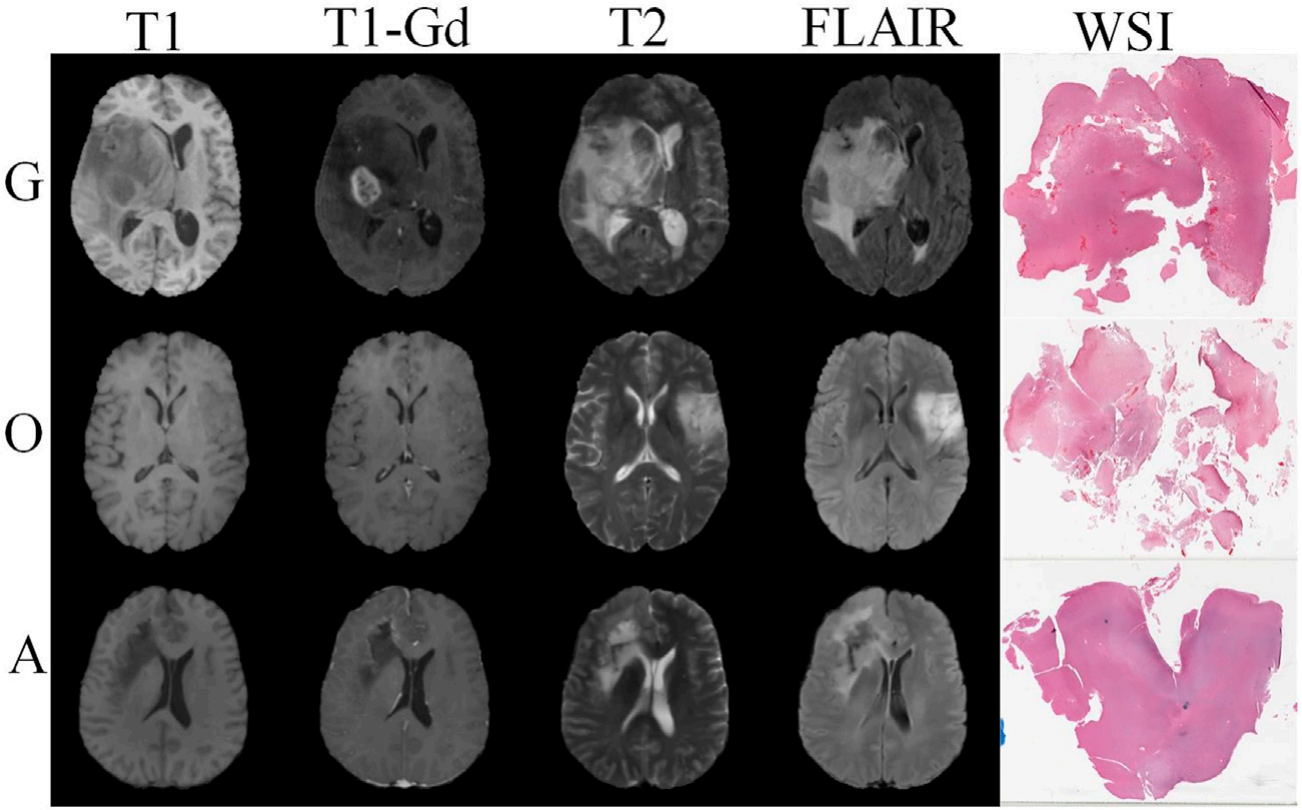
Visualization of glioblastoma (G), oligodendroglioma (O), and astrocytoma (A) in four sequences of MRI images and paired pathology images.
